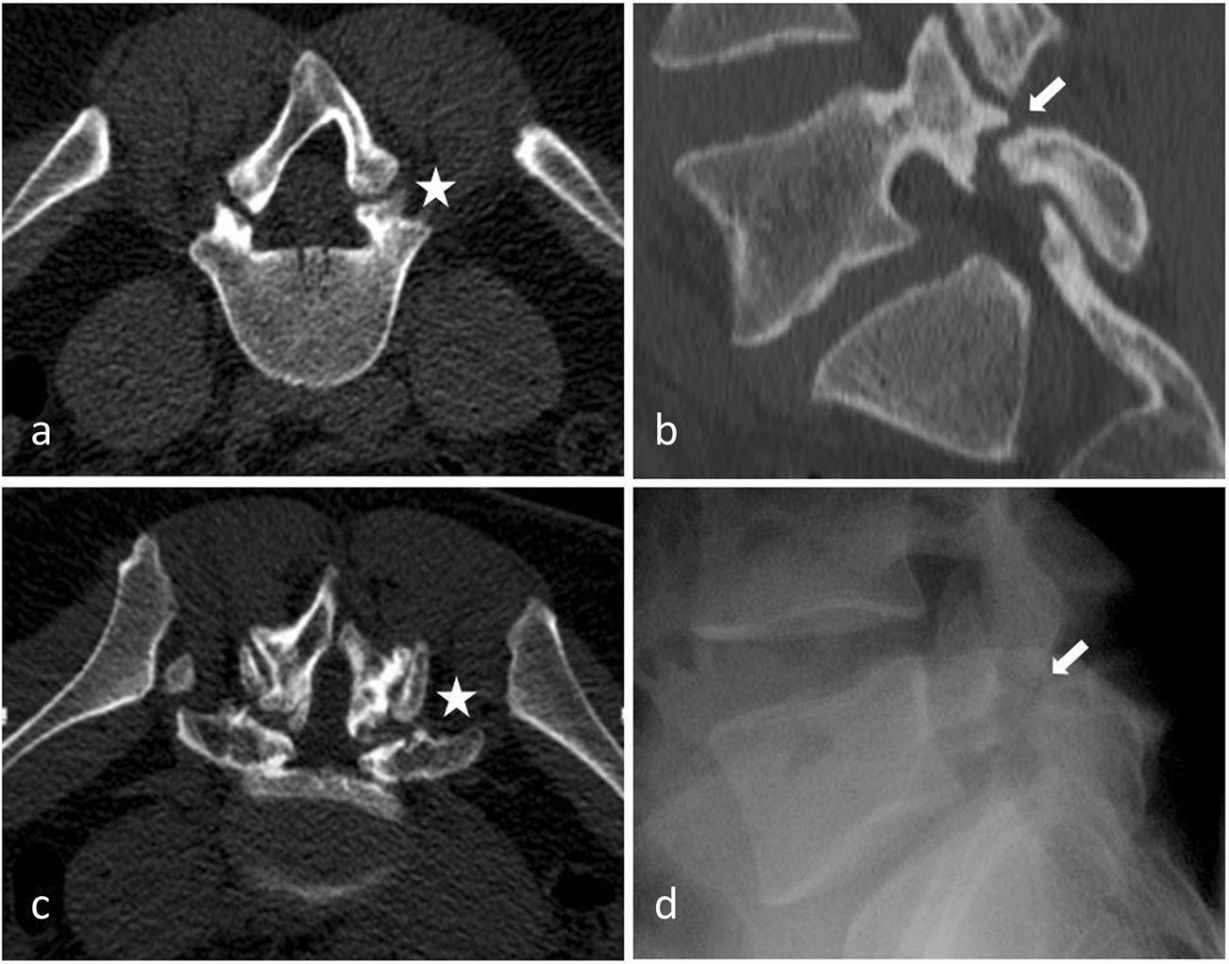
Isthmic lysis. a: Axial CT view at L4–5 level; b: axial CT view at L5-S1 level c: X-ray sagittal view at L5-S1 level; d: sagittal CT view L4–5 level
